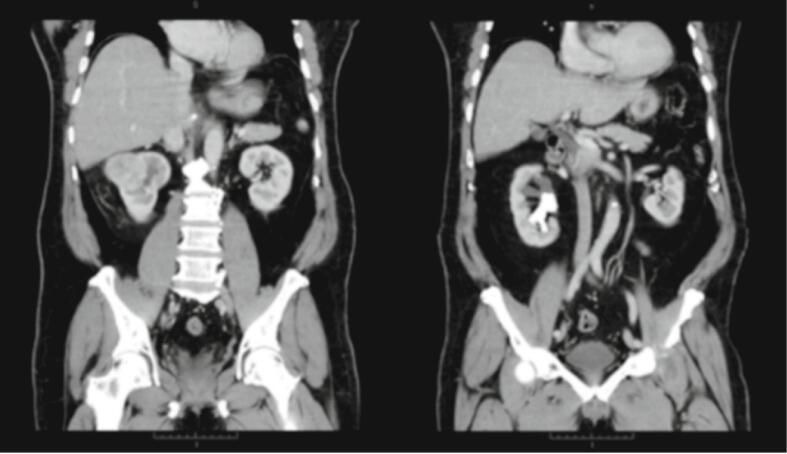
Abdominal CT scan with contrast showing staghorn stones and an inhomogeneous solid mass with contrast enhancement at the upper pole of the right kidney.
